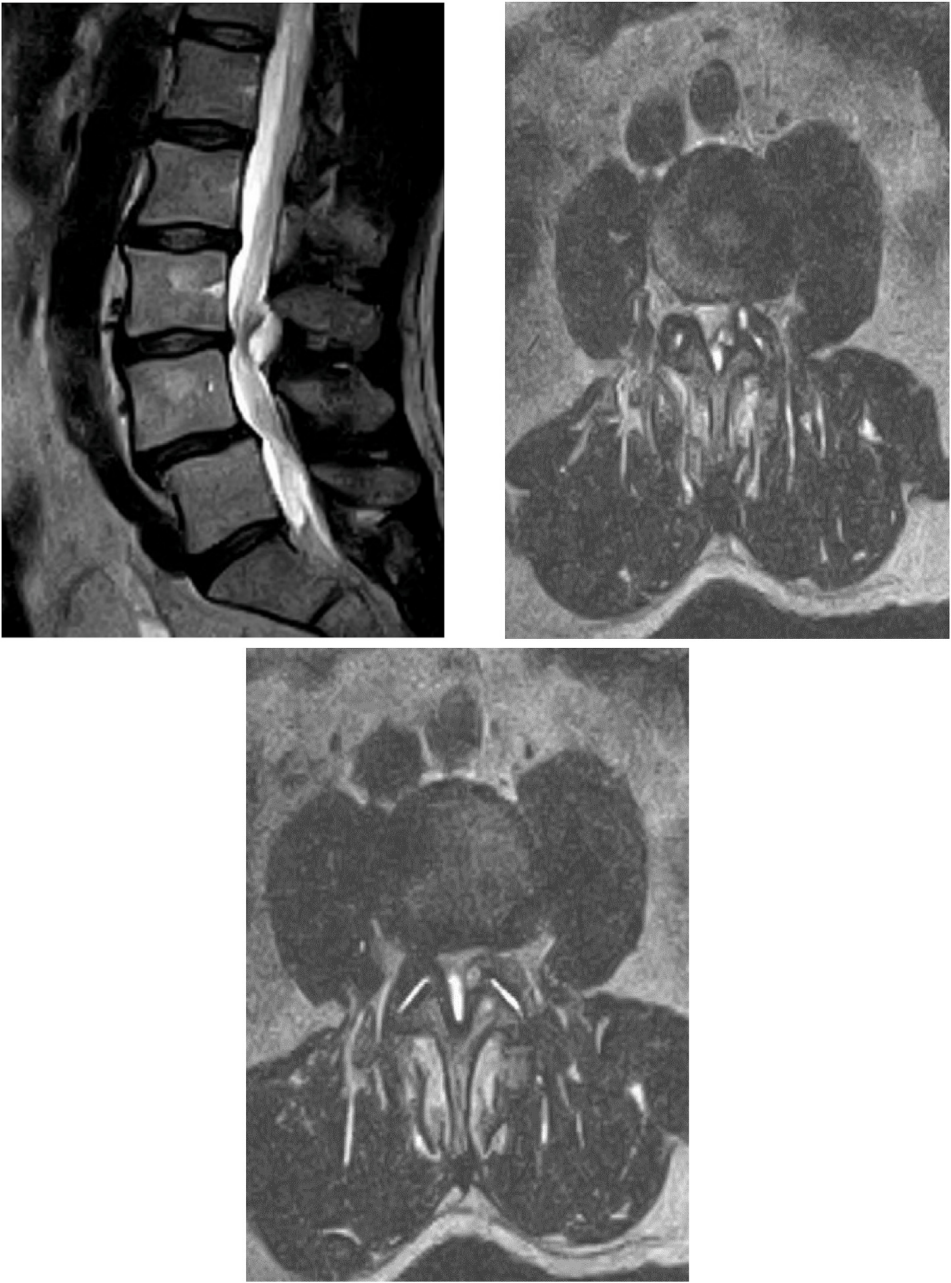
T2 sagittal and axial MRI demonstrating a complex left L3-4 juxtafacet cyst with resultant severe canal stenosis.
